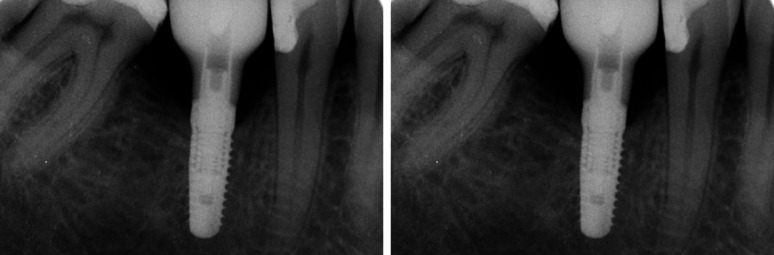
Radiographs of a ZLS-ISC at prosthetic delivery (left) and follow-up (right)
